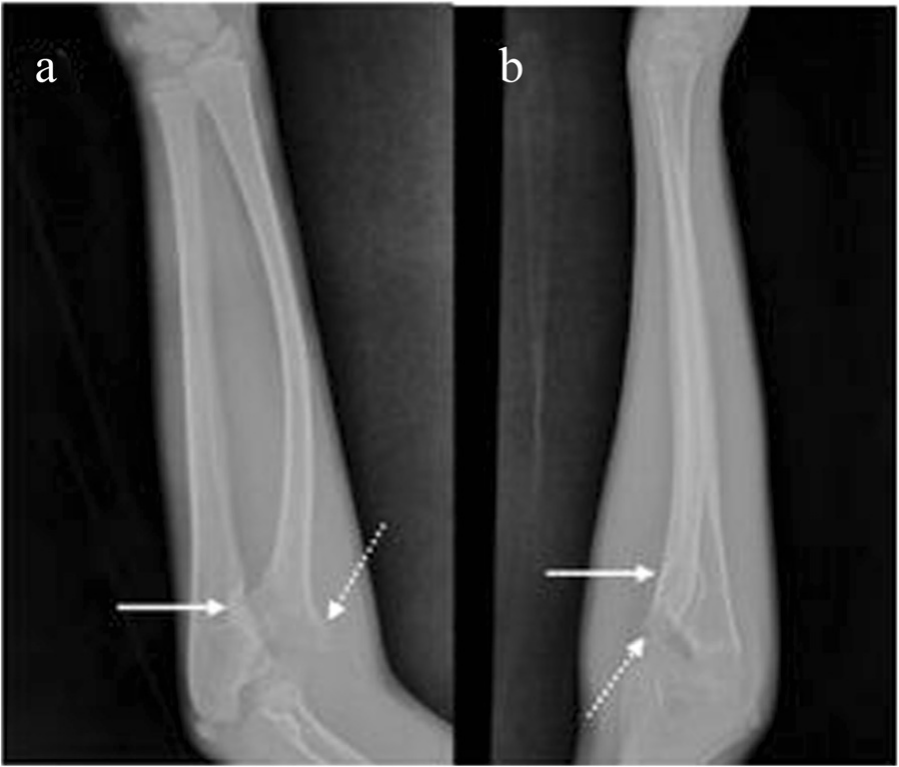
Preoperative radiographs. (a) Oblique and (b) anteroposterior radiographs of the right forearm showed a wide basal bony protuberance (solid arrow) in the dorsal metaphysis of the right proximal radius,and local depression with a dense edge;radial deformities in the proximal end, the radial shaft bending dorsally, ossification center shrinkage, and anterolateral dislocation (dotted arrow) were noted.
